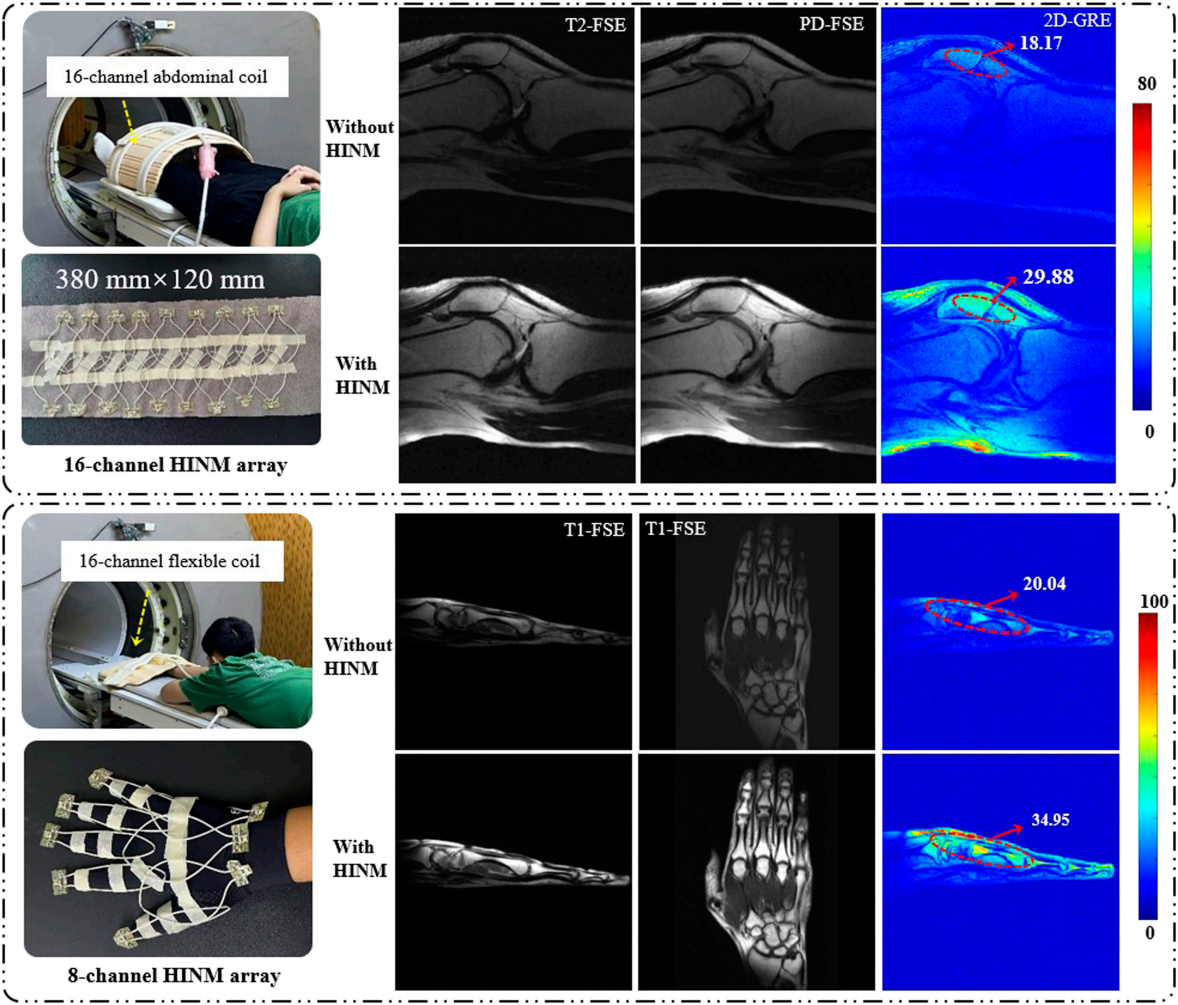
Low-field (0.5 T) MRI of the knee and hand using a commercial 16-channel abdomen coil and a 16-channel flexible coil with the HINM arrays.
